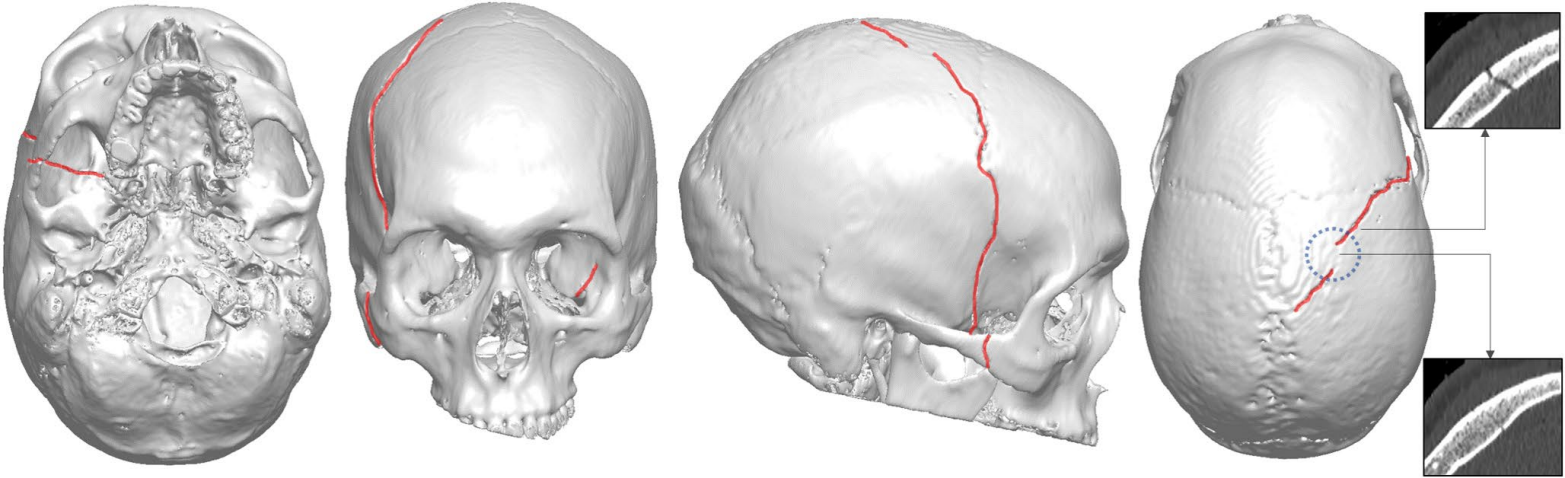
3D visualization of the victim’s skull bone seen from below, front, right and above. The fracture, highlighted in red, goes from the top of the head in the parietal bone towards the right side and continues along the skull base to the left sphenoidal sinus. Note the fracture on the right zygomatic bone and the interruption in the fracture, emphasized by the CT cross sections at two locations. The estimated region of impact is highlighted by the blue dotted circle on the top view of the cranium
